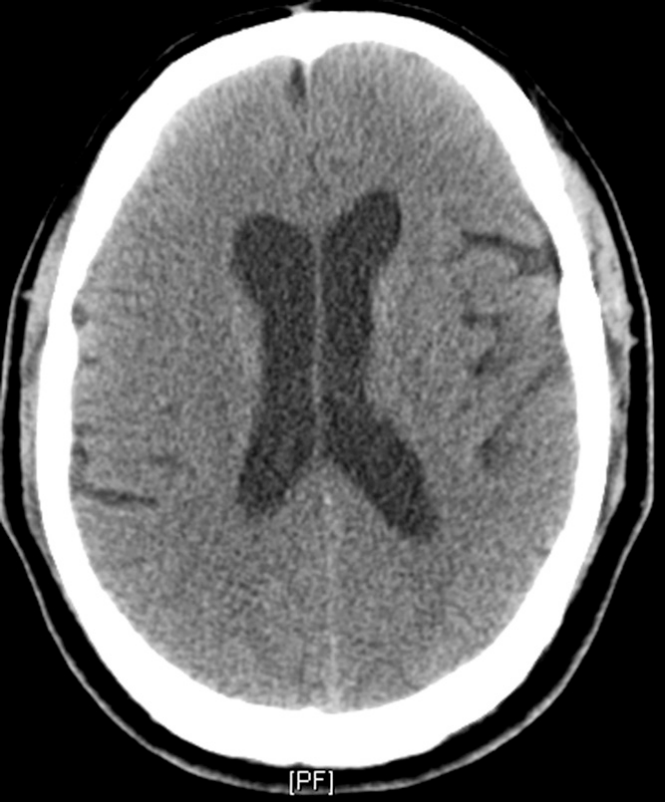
Computed tomography of the head of the patient without contrast status post removal of intraventricular cysticercal cysts.
